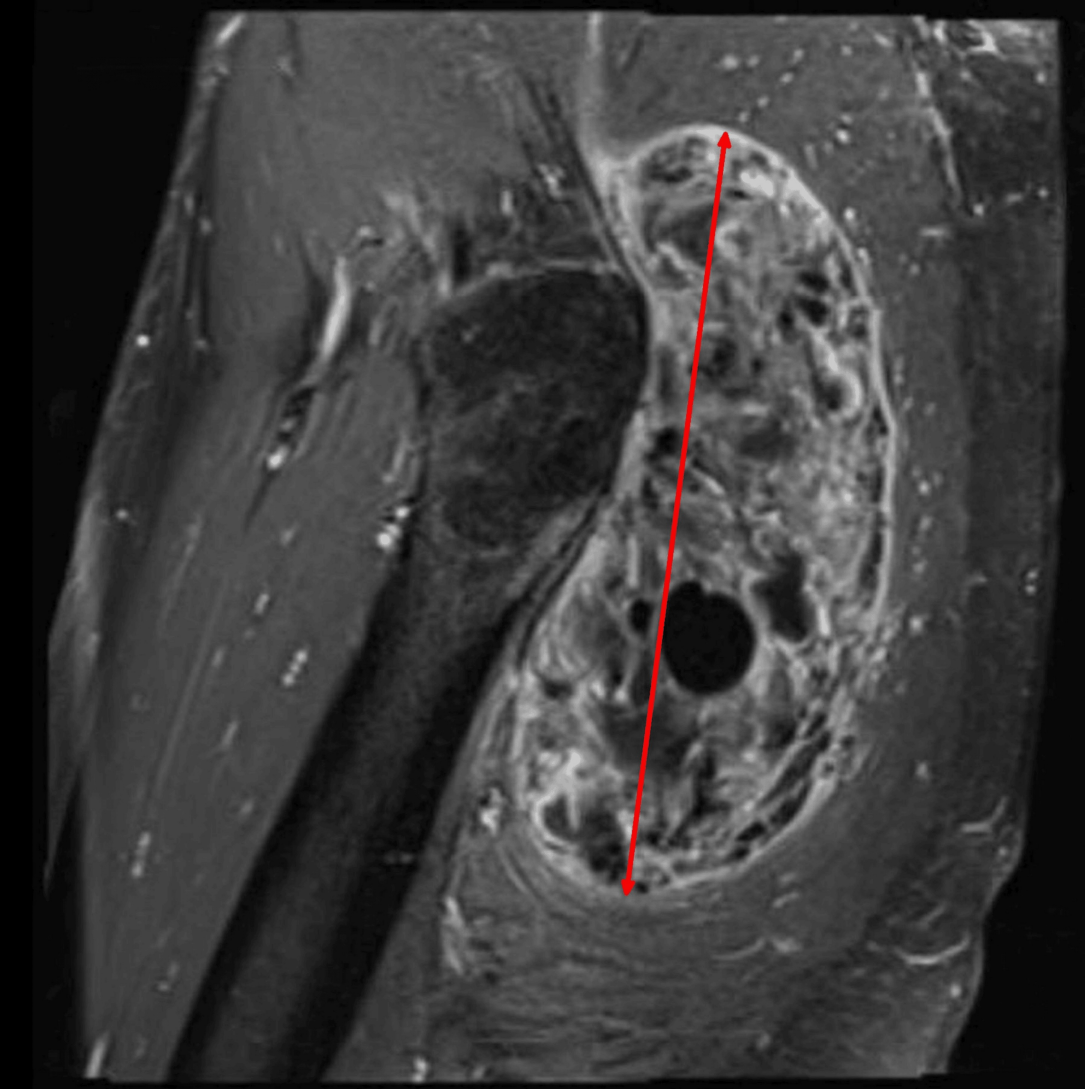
MRI hip: Sagittal view of the mass shown in Figure 2 The distance between the arrow heads is 15.7.
